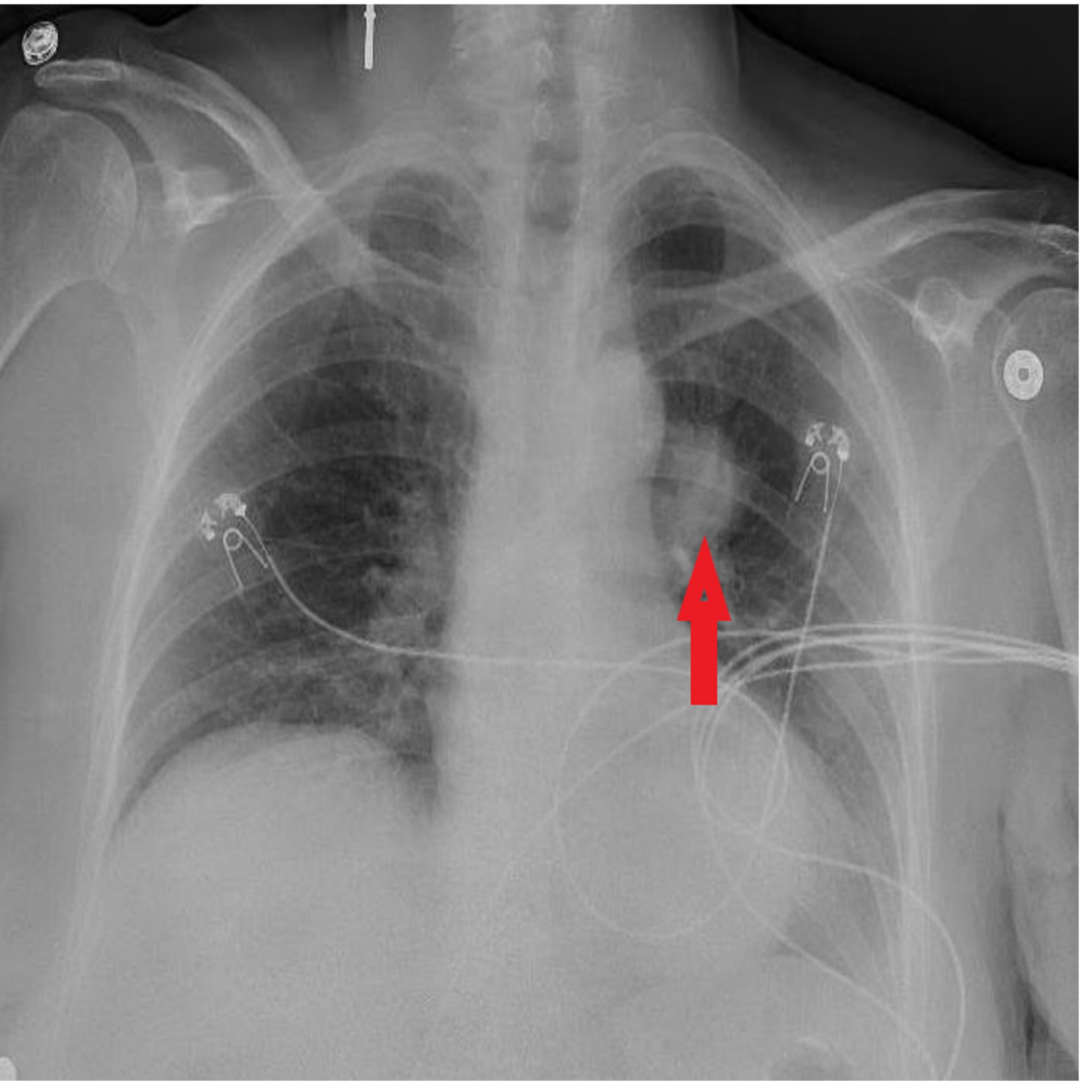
Chest X-ray showing round mass-like opacity in left suprahilar region
